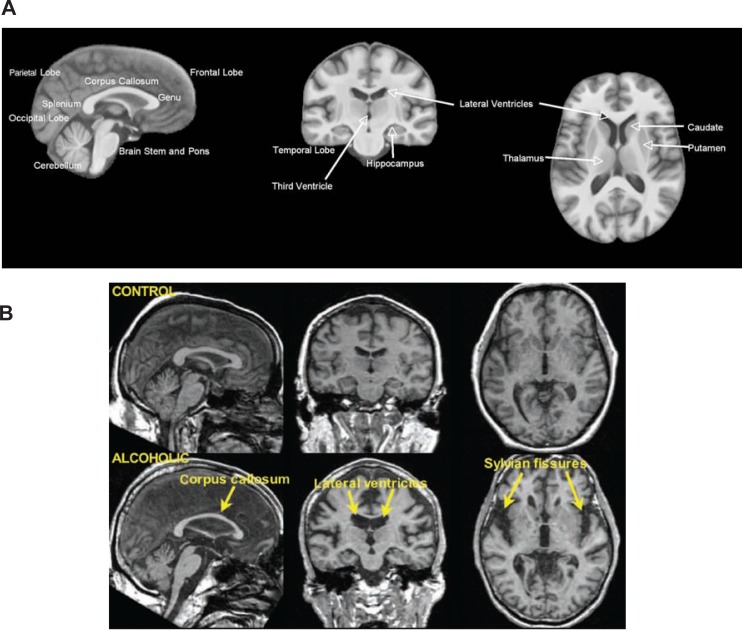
A) Standardized magnetic resonance imaging (MRI) of the brain viewed from the side, sagittal (left); back, coronal (middle); and above, axial (right). The dark areas represent fluid, white represents white matter, and shades of gray represent different gray matter areas and structures. Specific cortical regions and subcortical structures are labeled. B) MRI scans from a 53-year-old control man (upper) and a 53-year-old alcoholic man (lower) from the same views as shown above. Note the enlargement of the lateral ventricles and sulci, reduced cortical tissue, and skinnier corpus callosum in the alcoholic compared with the control.
